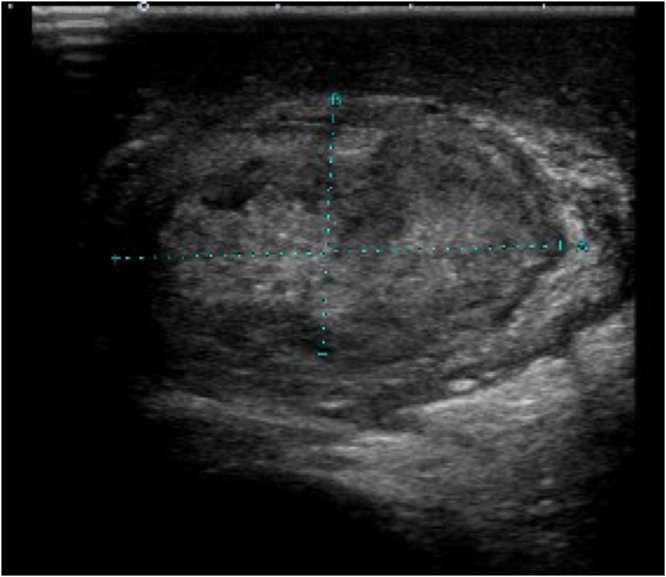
Scrotal ultrasound showing hypoechoic testis without testicular torsion.
